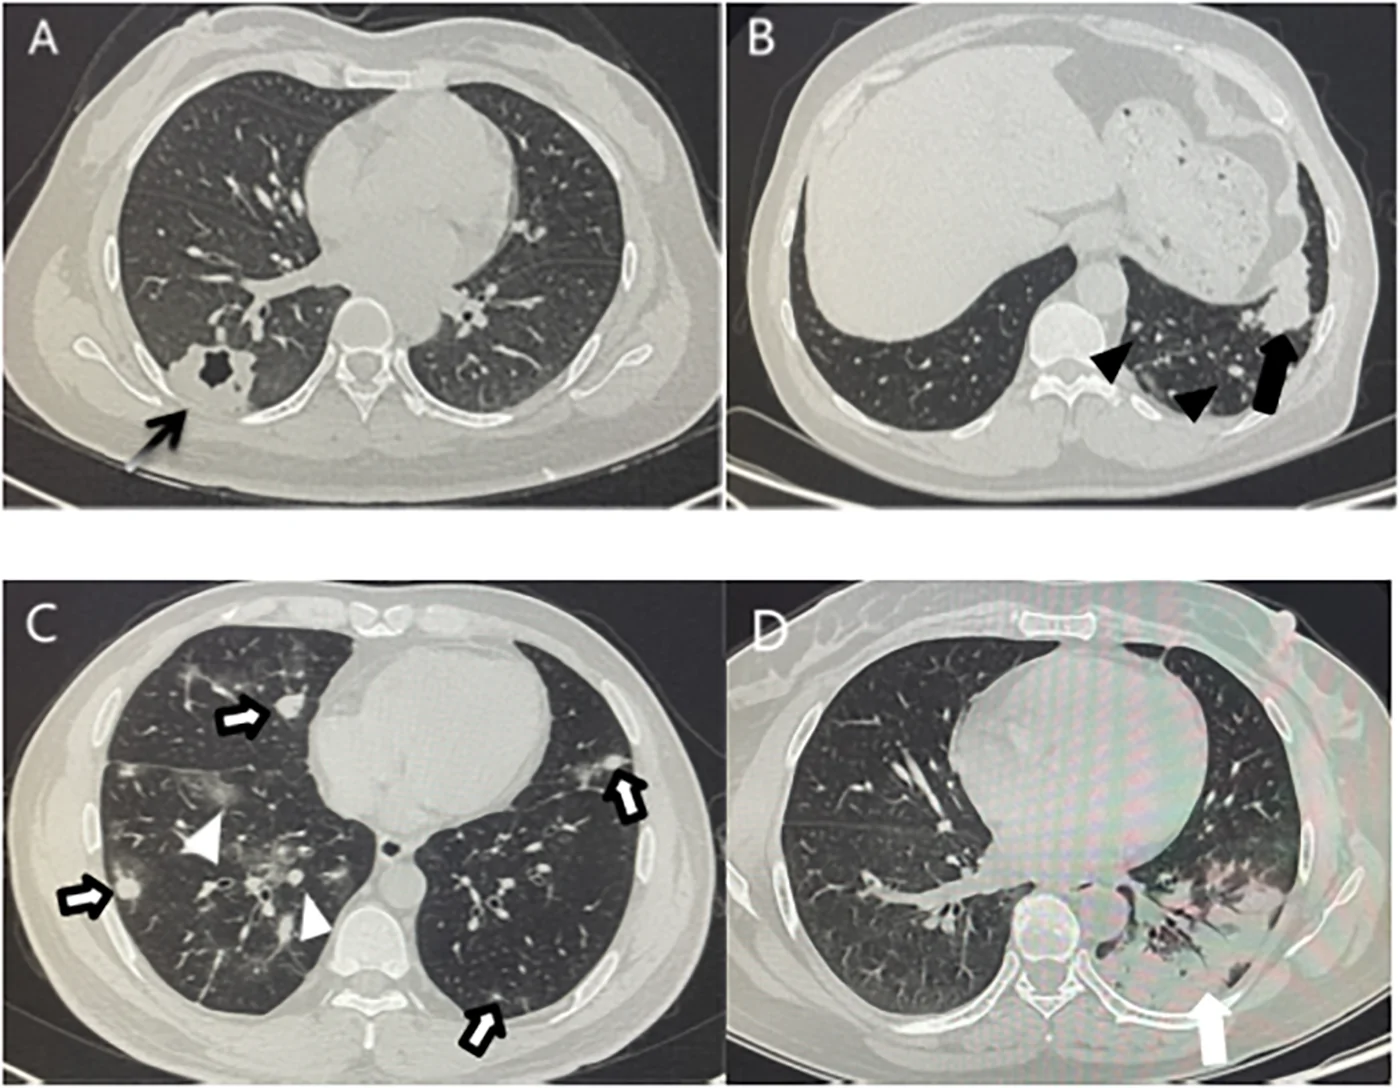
Representative chest CT images of pulmonary cryptococcosis. (A) Solitary nodule/mass type: A lobulated mass with internal cavitation (black arrow) in the right lower lobe. (B) Multiple nodules/masses type: Multiple irregular nodules (black arrowheads) and masses (black arrow) in the left lower lobe. (C) Mixed type: Multiple patches (white arrowheads) and nodules (black hollow arrows) distributed in both lungs, with some nodules surrounded by halo signs. (D) Patchy consolidation type: A large consolidation with air bronchogram (white arrow) in the left lower lobe.
